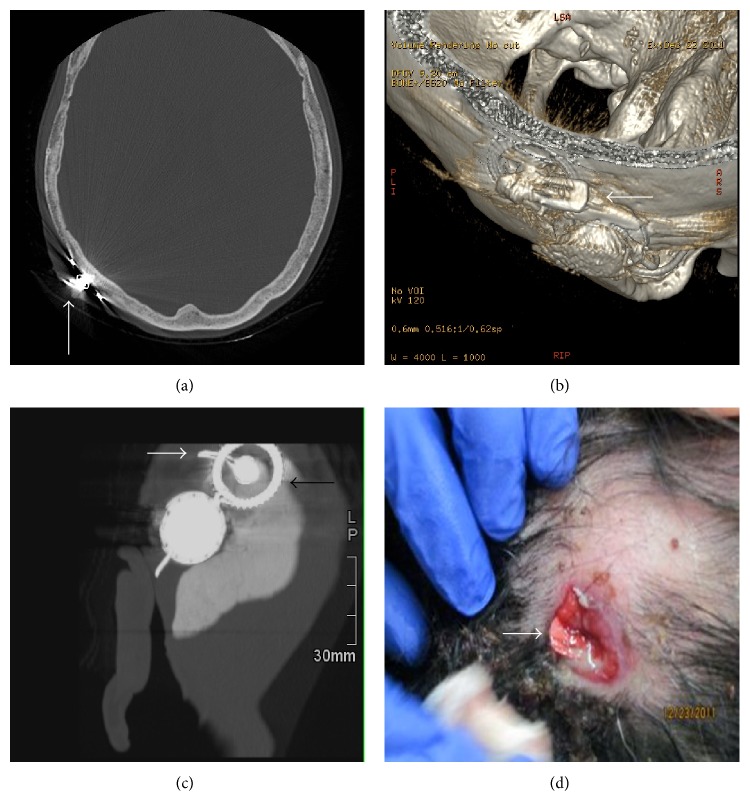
CT images ((a) axial view, (b) multiplanar reconstruction with maximum-intensity projection, and (c) 3D volume rendering) and (d) intraoperative findings of the barrette hair clip (white arrows) and its spatial relationship with the magnet of the receiver stimulator (black arrow).
